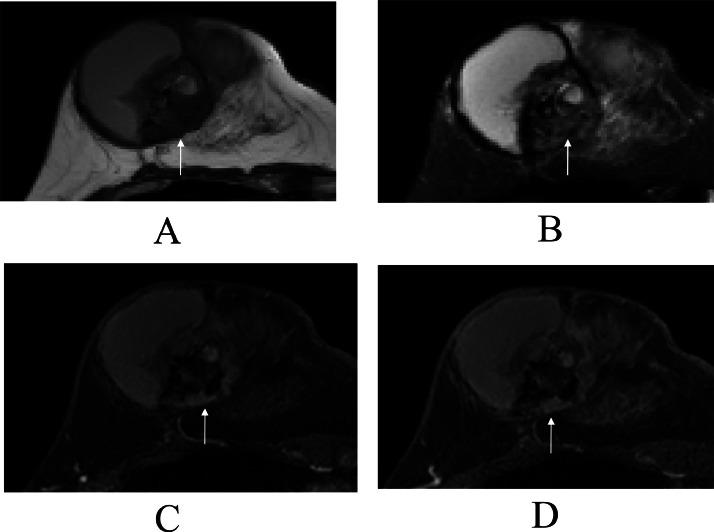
Magnetic resonance imaging (MRI) findings. MRI of the mass components (arrow) showed a hypo intense pattern on T1-weighted images (A) and a mosaic pattern on T2-weighted images (B). Enhanced MRI of the mass components (arrow) showed a faint enhancement pattern both on early (C) and late (D) phase images.
